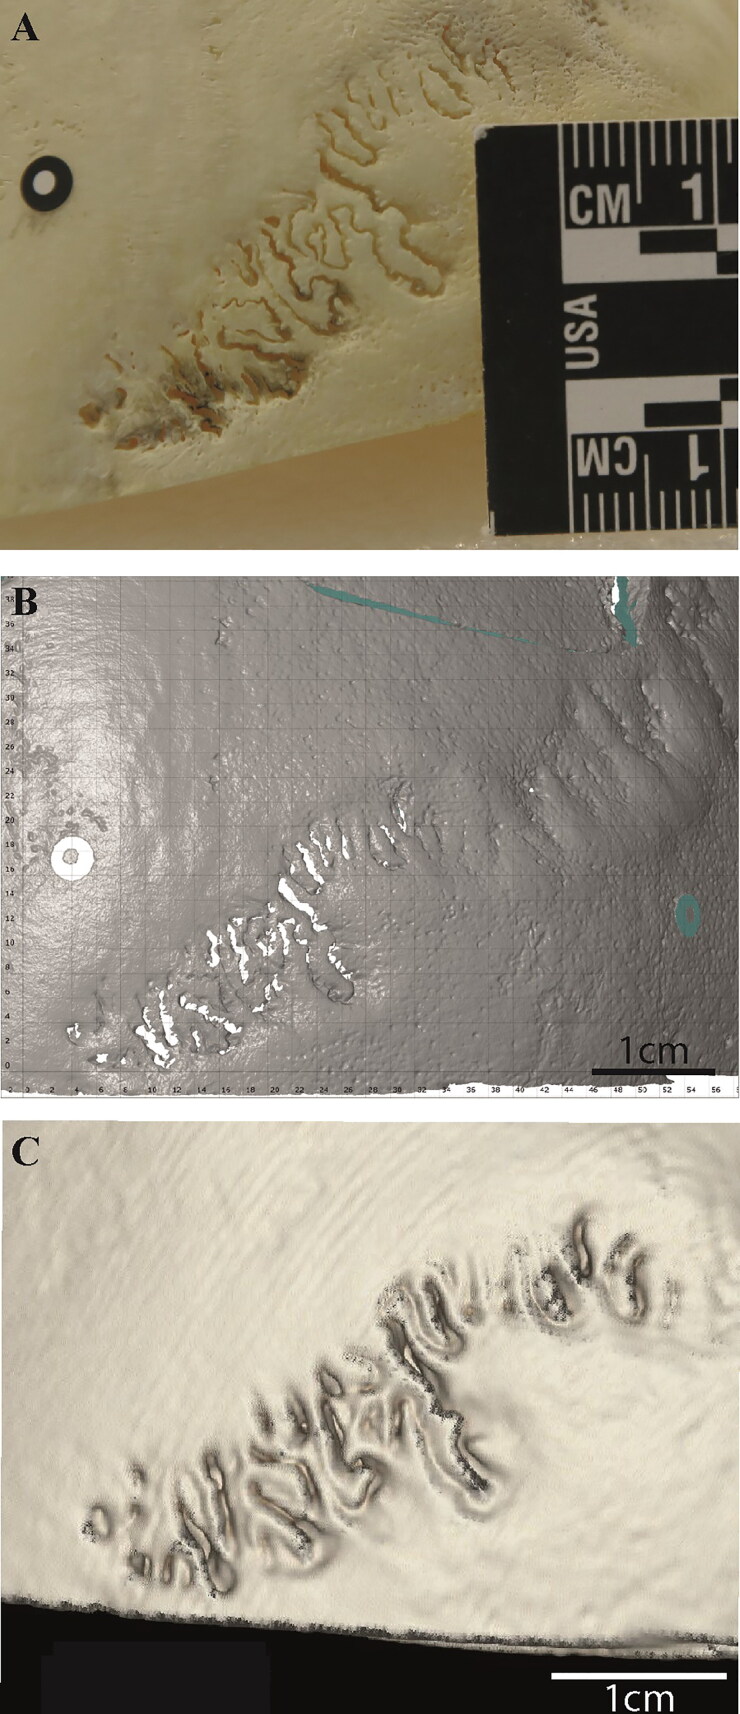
Comparison between macroscopic (A), 3D surface scanning (B), and CT model (C) of a left midlamboid suture. In the CT model (C), the edges of the sutures appear closer together, “rounded”, and less complex than in the dry bone observation. This image demonstrates the shift in perception between three observation media. In A and C, the scale is in cm; in B, it is in mm.
